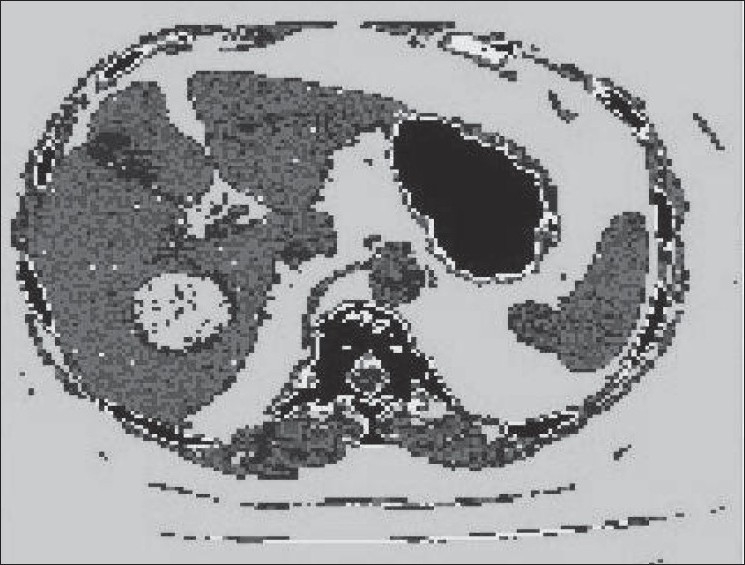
Segmentation of Abdomen (CT image using region based technique)
